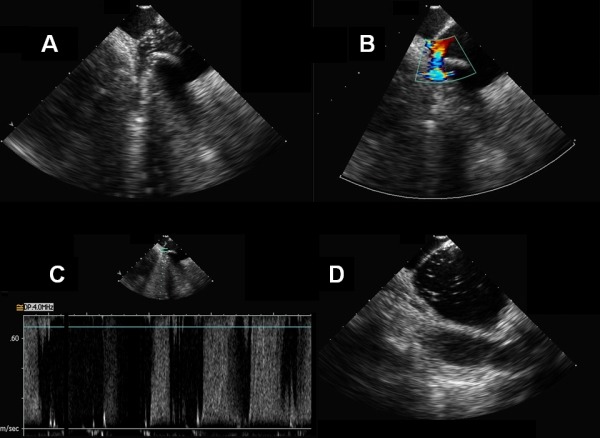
Contrast and Doppler intracardiac echocardiography: absence of occlusion. A. Contrast intracardiac echocardiographic view showing an inferior gap between right inferior pulmonary vein and the cryoballoon. By injecting saline solution through the balloon catheter central lumen, a back-flow of microbubbles is observed due to periballoon leak. B. Color flow Doppler visualization of turbulent leakage flow in the same pulmonary vein. C. Pulsed wave Doppler pattern of high-frequency monophasic leakage flow at the same anatomical gap. D. Same patient, left superior pulmonary vein: straightforward echocontrastographic effect of microbubbles back-flow into the left atrium after saline solution injection into the targeted pulmonary vein, despite the difficulty in analyzing the whole region of PV antrum by Doppler echocardiography.
